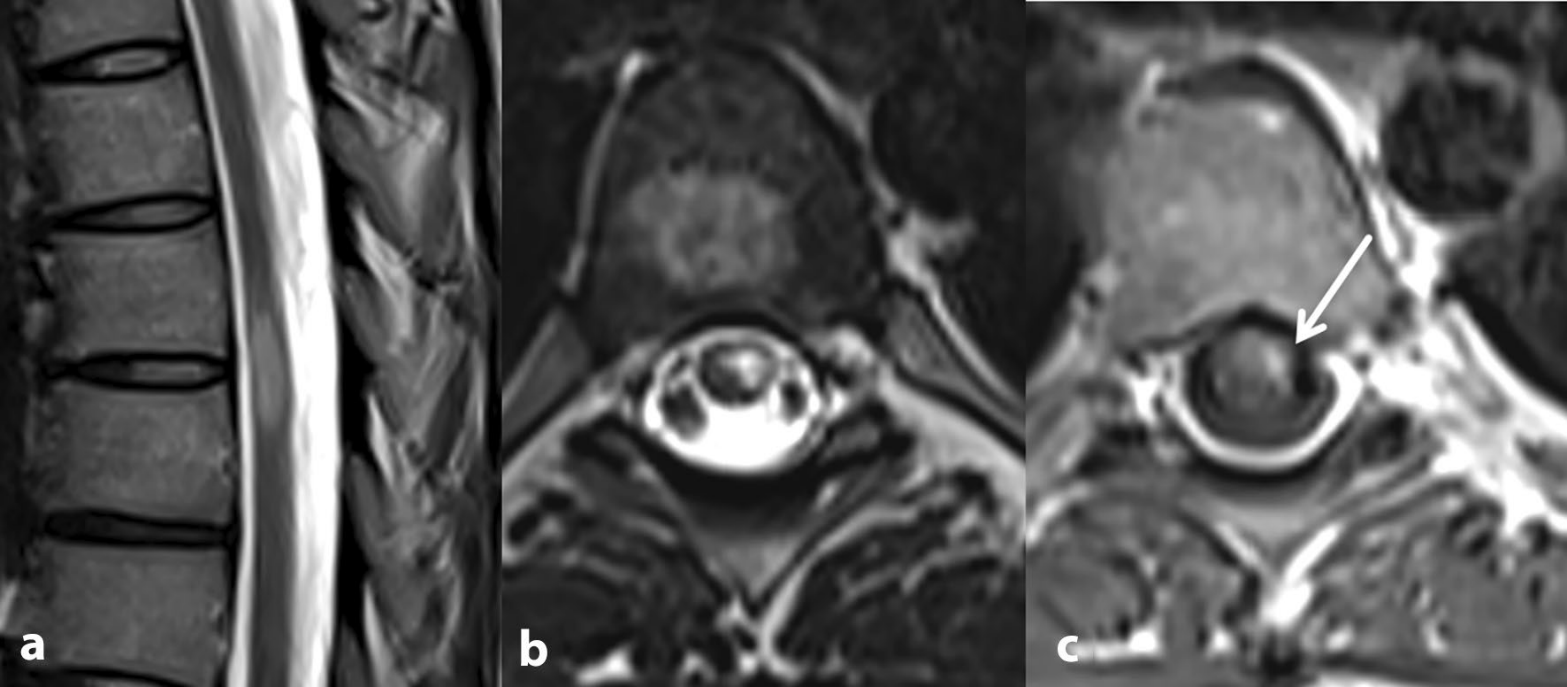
Spinal MRI: a, b peripherally located, T2 hyperintense lesion at level T6 and T7. The craniocaudal extension is less than two vertebral body segments. c Contrast enhancement after application of gadolinium is consistent with an active lesion. Thus, the criteria of spatial dissemination are fullfilled
